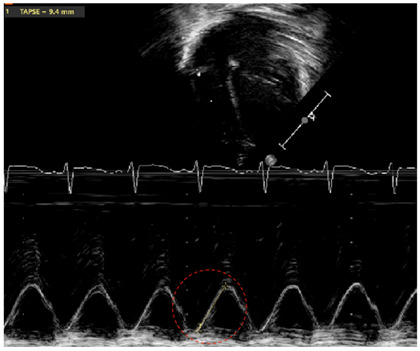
Legend: Marker of longitudinal systolic function of the right ventricle (RV) (which primarily contracts longitudinally). The M(motion)-Mode is used with the line of interrogation passing through the attachment of the tricuspid valve at the level of the free wall of the RV, and through the RV apex. The distance travelled from end-diastole to peak of systole is measured by following the line of the attachment of the tricuspid valve on the M-Mode tracing through time (red circle). Occasionally, superimposed tissue Doppler allows to follow the period of systole with increased precision. Z-scores have been published by gestational age/postmenstrual age, as well as chronological age for term infants [34,56].
